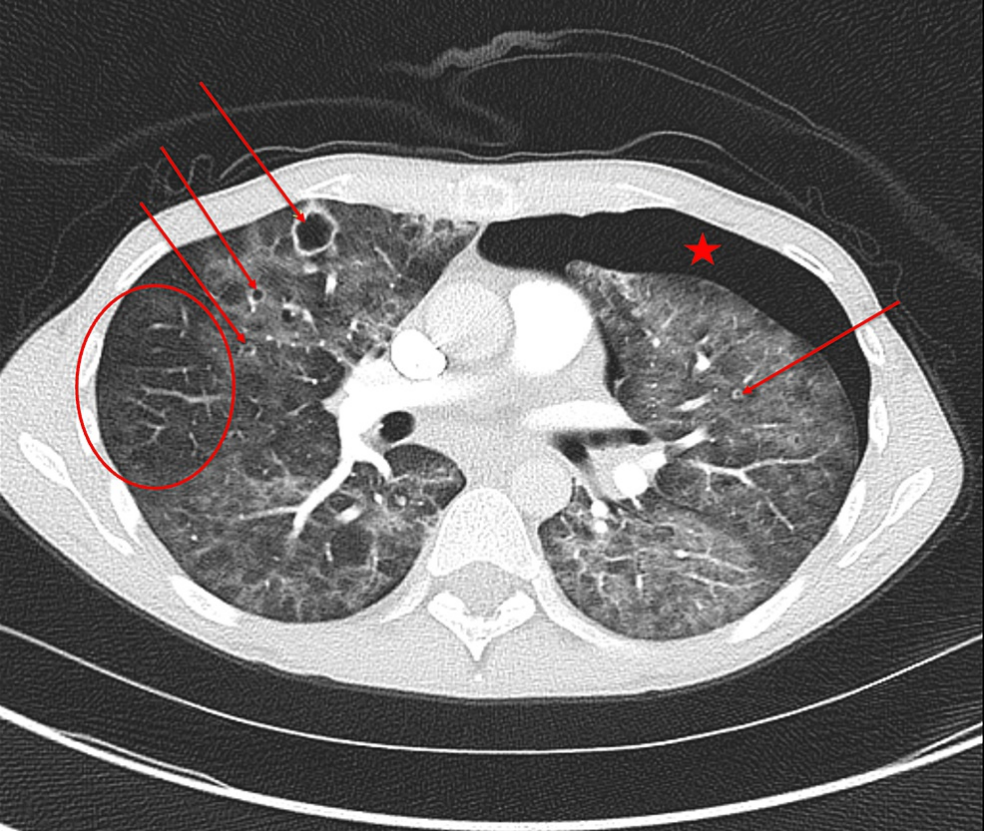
Chest CT axial section demonstrating bilateral diffuse ground-glass opacities, left pneumothorax (red star), subpleural sparing located in the right superior lobe (red circle), and multiple bilateral pneumatoceles located in the anterior segment of the right superior lobe and the anterior segment of the left superior lobe (red arrows).
